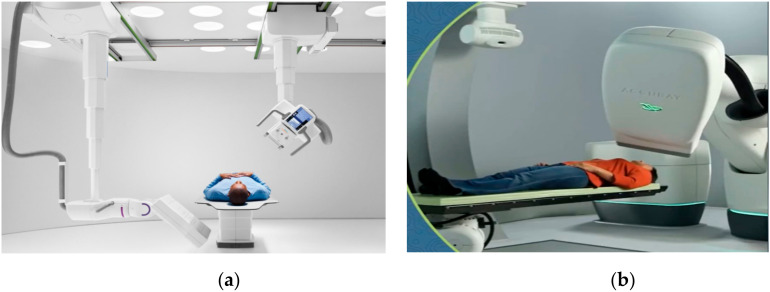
Specialized robots for spine and cranial biopsy and surgery. (a) Multitom Rax: Twin Robotic X-ray from Siemens Healthineers [65]. (b) Cyberknife: Radiology Oncology Surgical robot [66].
